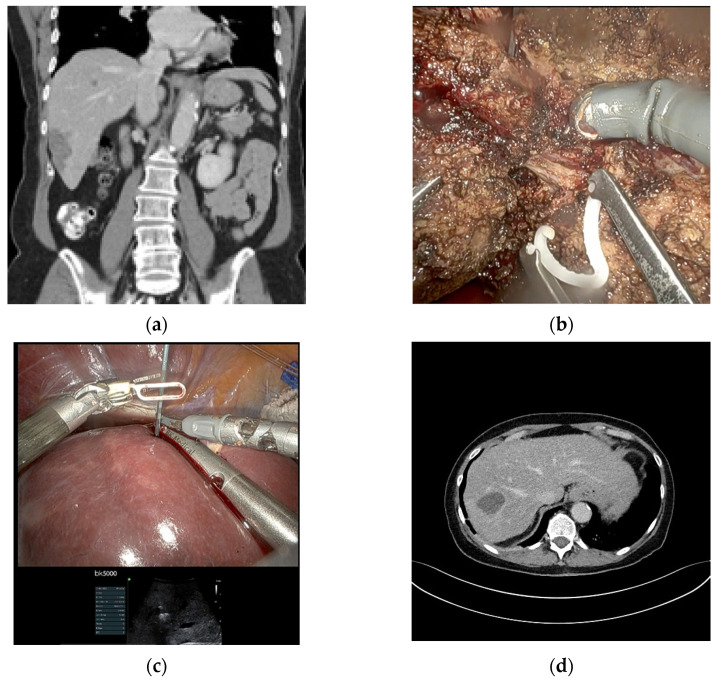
Patient with an AE lesion in S VI and a second small lesion in S VIII, who underwent anatomic S VI resection + radiofrequency ablation of the lesion in S VIII. (a) Preoperative CT scan (coronal view); (b) Intraoperative image showing the central ligation of the segmental portal vein branch to S VI; (c) Intraoperative image showing the ultrasound-guided radiofrequency ablation of the lesion in S VIII; (d) Postoperative CT scan showing the ablation result (transversal view).
